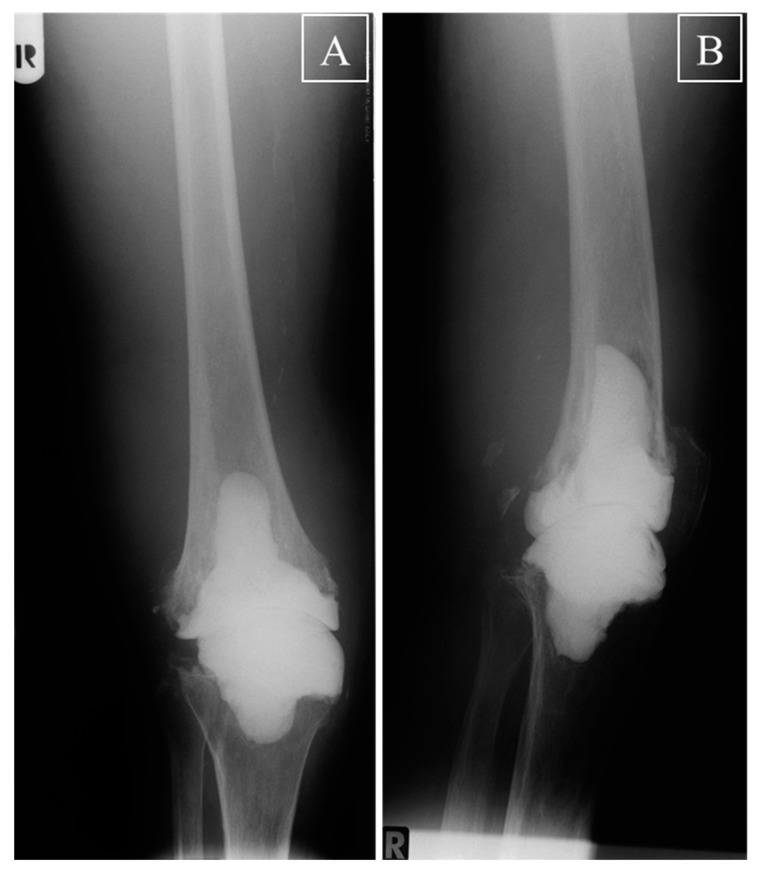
Knee X-ray before re-implantation. (A) Anterior to posterior and (B) lateral X-ray of a right knee with the inverse spacer of one representative patient before re-implantation TKA. A perfect fit of the two different spacer components into the tibial and femoral medulla was observed, without any signs of dislocation, subluxation, fractures of spacer or bone, or increased gross abrasion of bone in the joint area; R = right.
